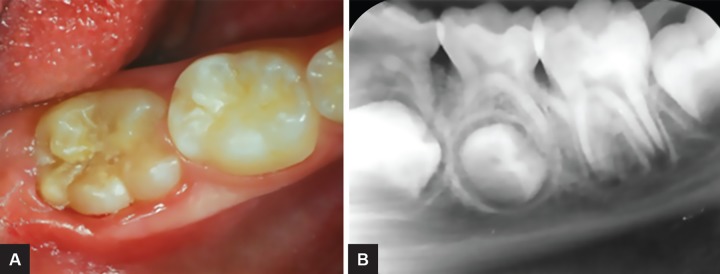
(A) Left mandibular first permanent molar partially erupted 6 months after surgical intervention. (B) Radiograph image of the first permanent molar 6 months after surgical intervention
